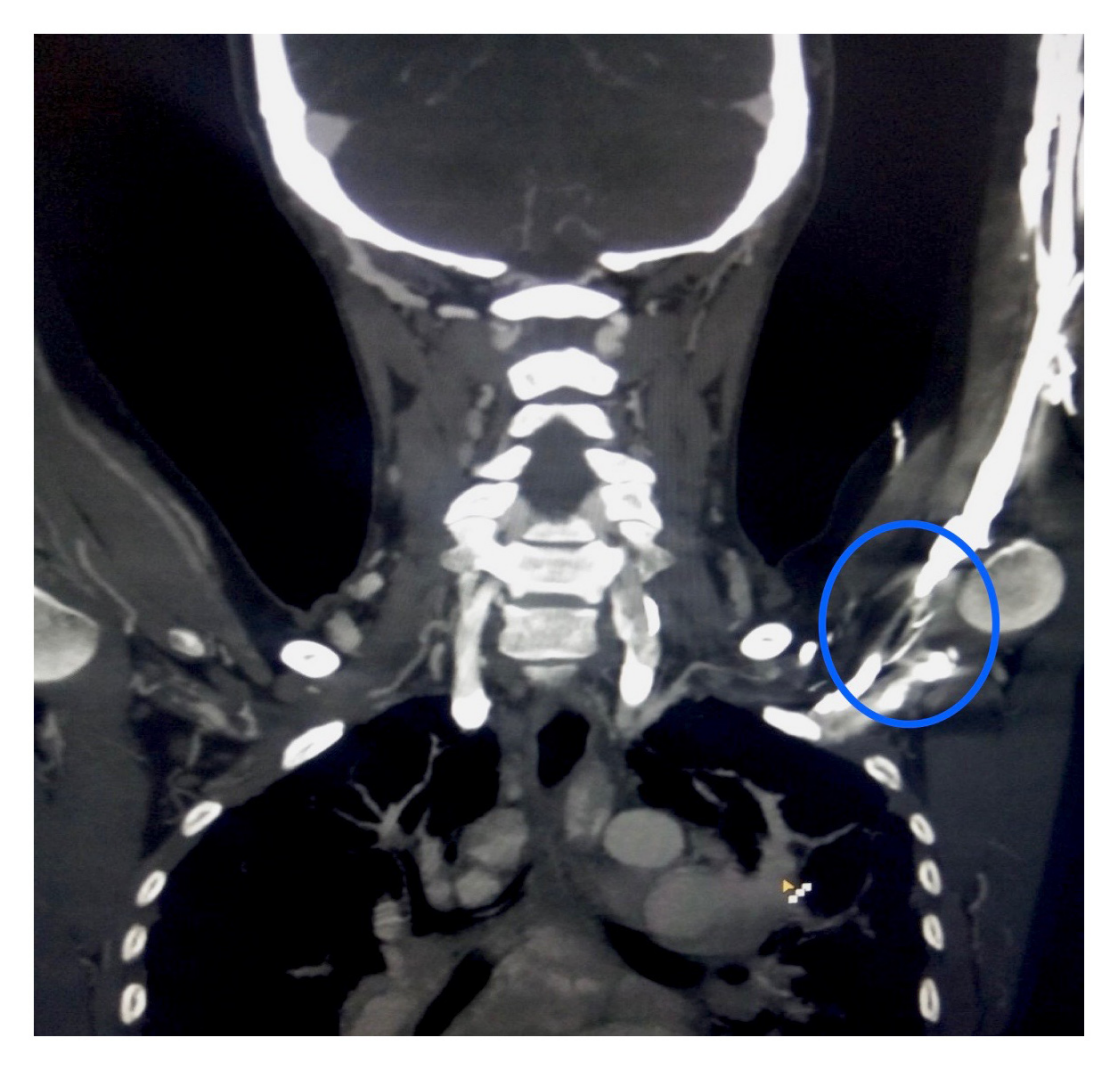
Computed tomography (CT) aortogram showing stenosis of left subclavian artery with almost complete block at distal part and proximal left axillary artery.
